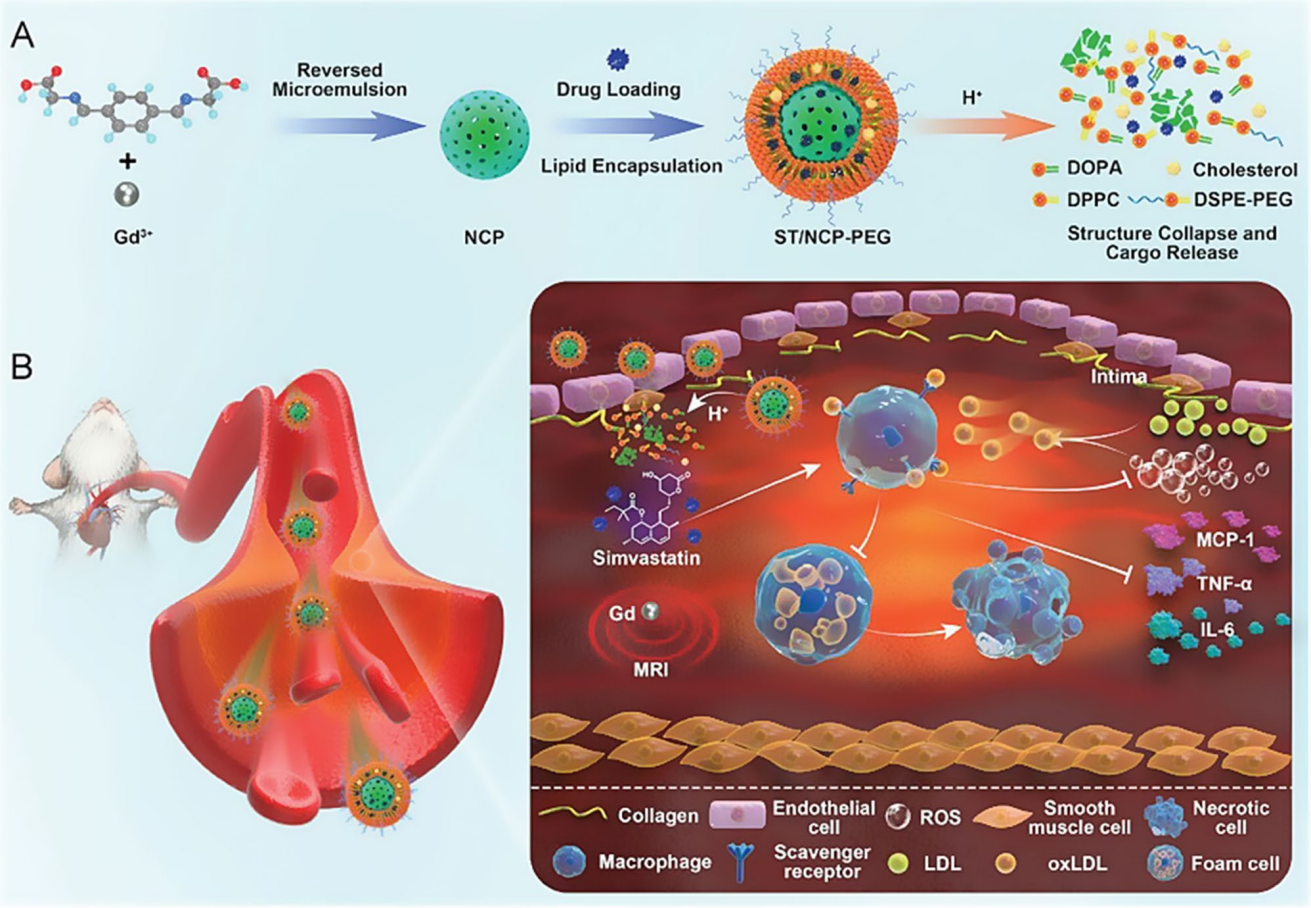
Design strategies for pH-responsive nanoplatforms. (A) the fabrication procedure of ST/NCP-PEG and pH-responsive structural collapse under acidic microenvironment. (B) the mechanism of pH-responsive and MRI-functional ST/NCP-PEG nanomedicine enabling spontaneous diagnosis and treatment of atherosclerosis. Adapted with permission from [80], copyright 2024, Wiley-VCH Verlag
